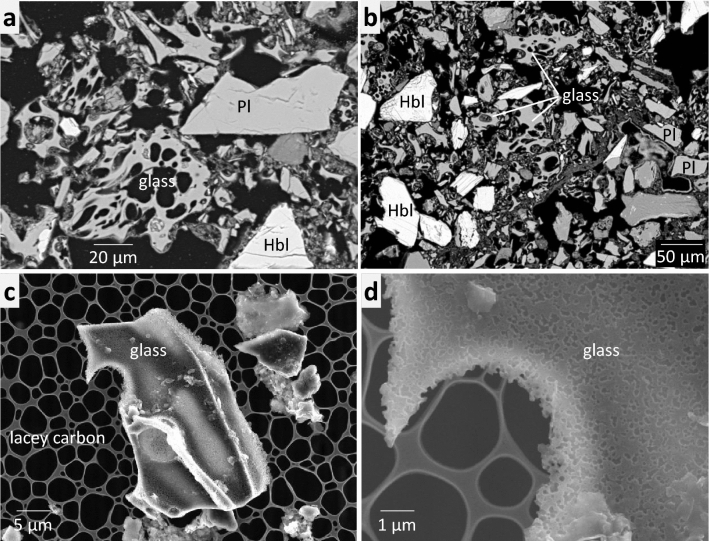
Morphology of volcanic glass. (a,b) Back-scattered electron images of thin section containing glass. (c,d) Secondary electron images of glass dispersed on a lacey carbon after ultrasonification. Pl: plagioclase, Hbl: hornblende, Qz: quartz.
